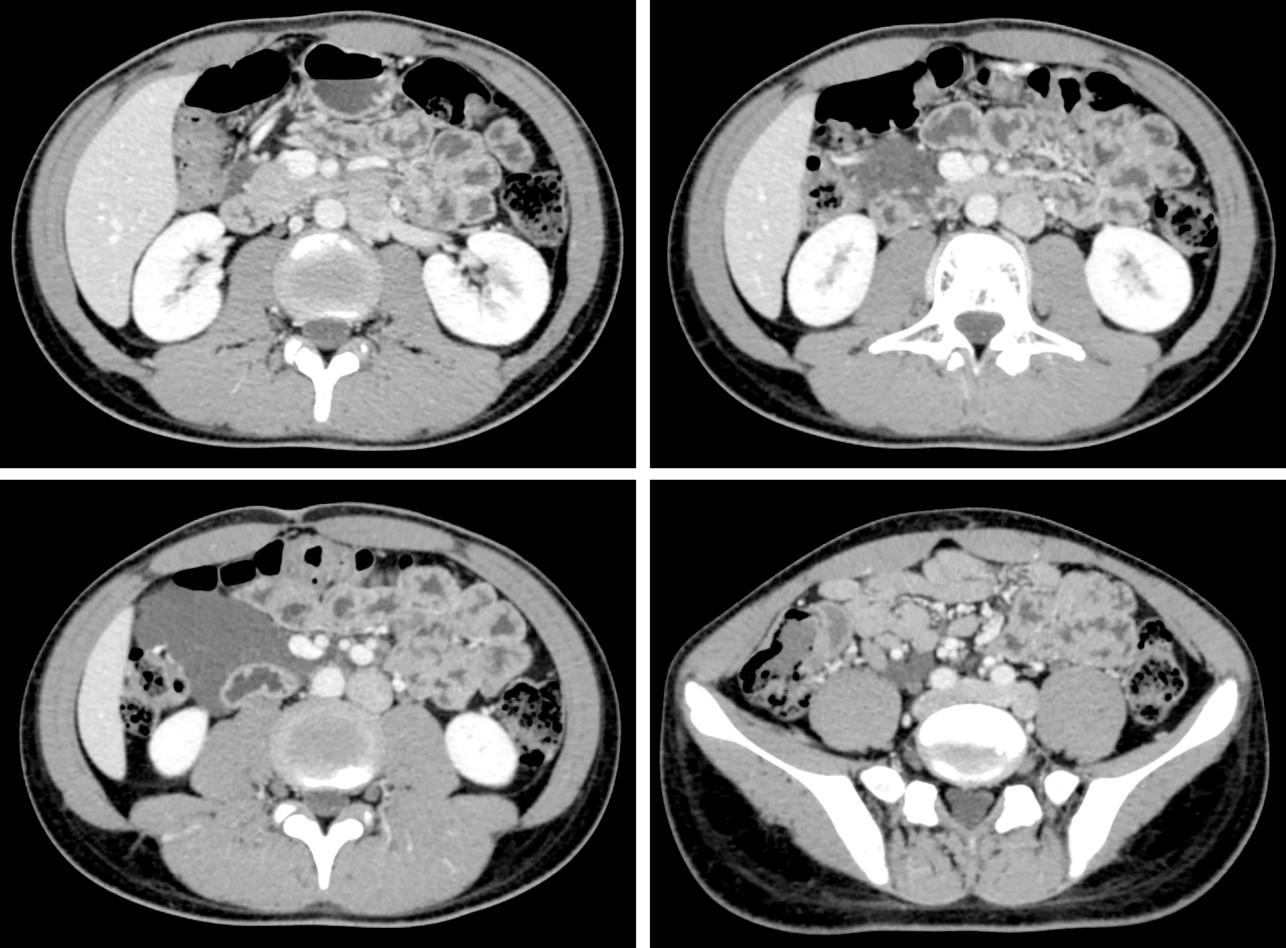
Computed tomography showed a large cystic mass.
